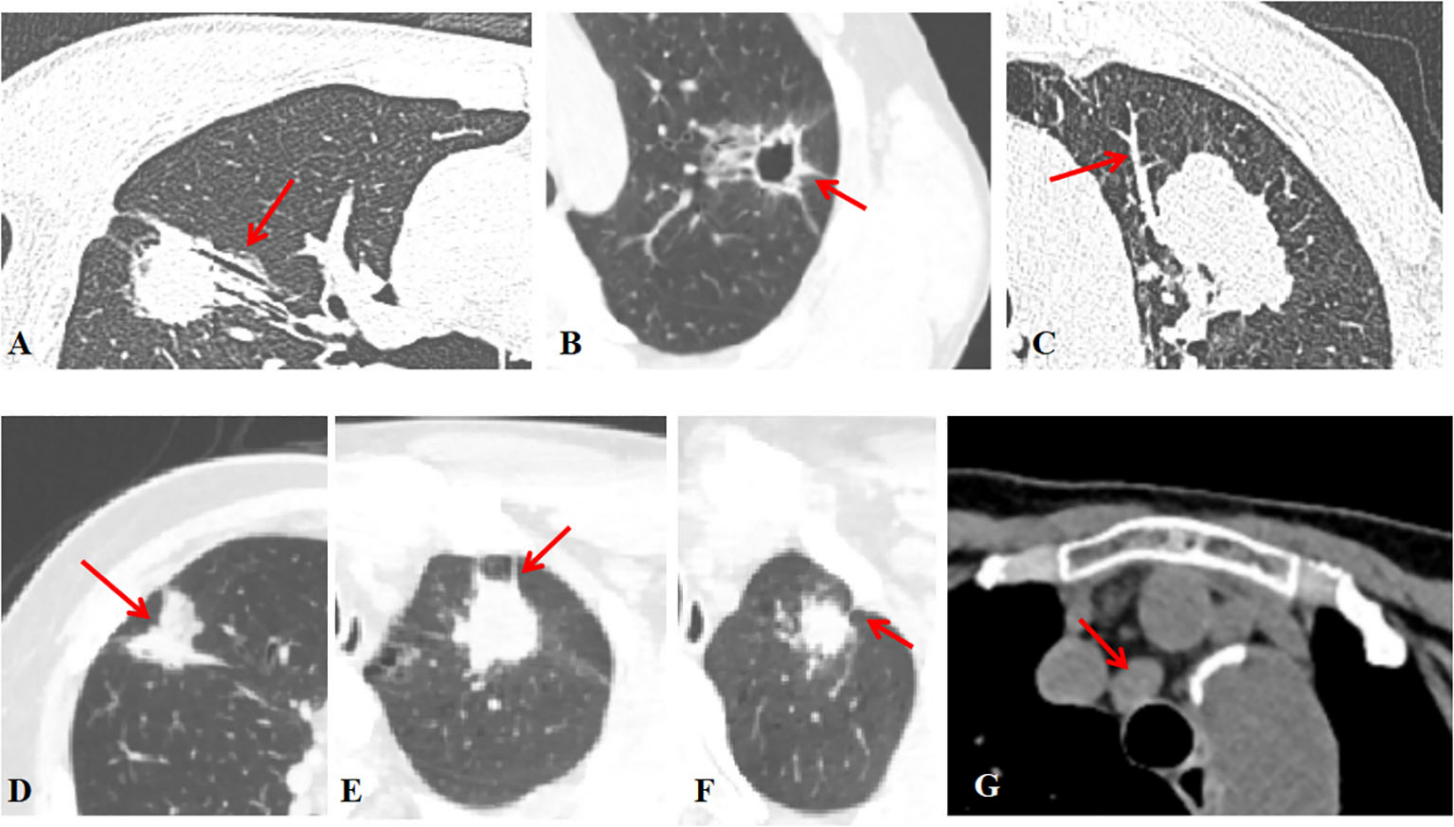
Radiographic features (A) The air bronchogram is fluid-filled or solid alveoli surrounded by air-filled bronchus. (B) The cavity is a gas-filled space >3 mm within a solid lung lesion, mass or nodule. (C) Vessel convergence sign was defined as an increase in internal vessels or clustering of vessels. (D) Lobulation was defined as a nodal margin showing an uneven lobar outline. (E) Spiculation was defined as a small spine-like protrusion from the nodule margin extending into the lung parenchyma without reaching the pleural surface. (F) Pleural indentation was defined as a tapered or linear extension of the lesion into the pleura, reflecting pulmonary fibrosis with retraction of the adjacent pleura. (G) Hilar and mediastinal lymph nodes were considered positive if the short axis exceeded 10 mm on chest CT images.
